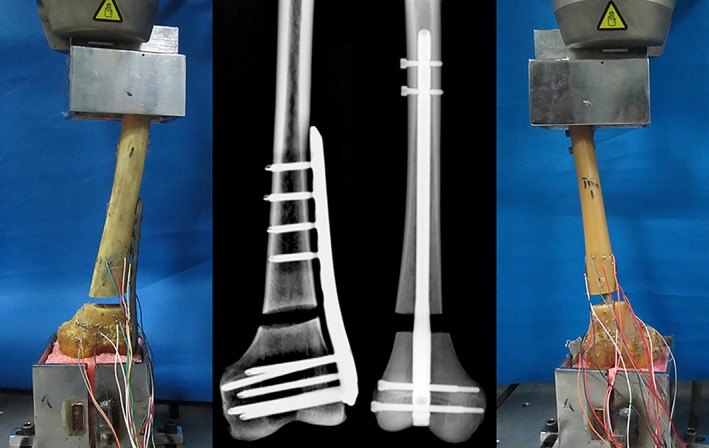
Radiographs of internal fixation of the distal femur and biomechanical loading experiment on Instron testing machine.
